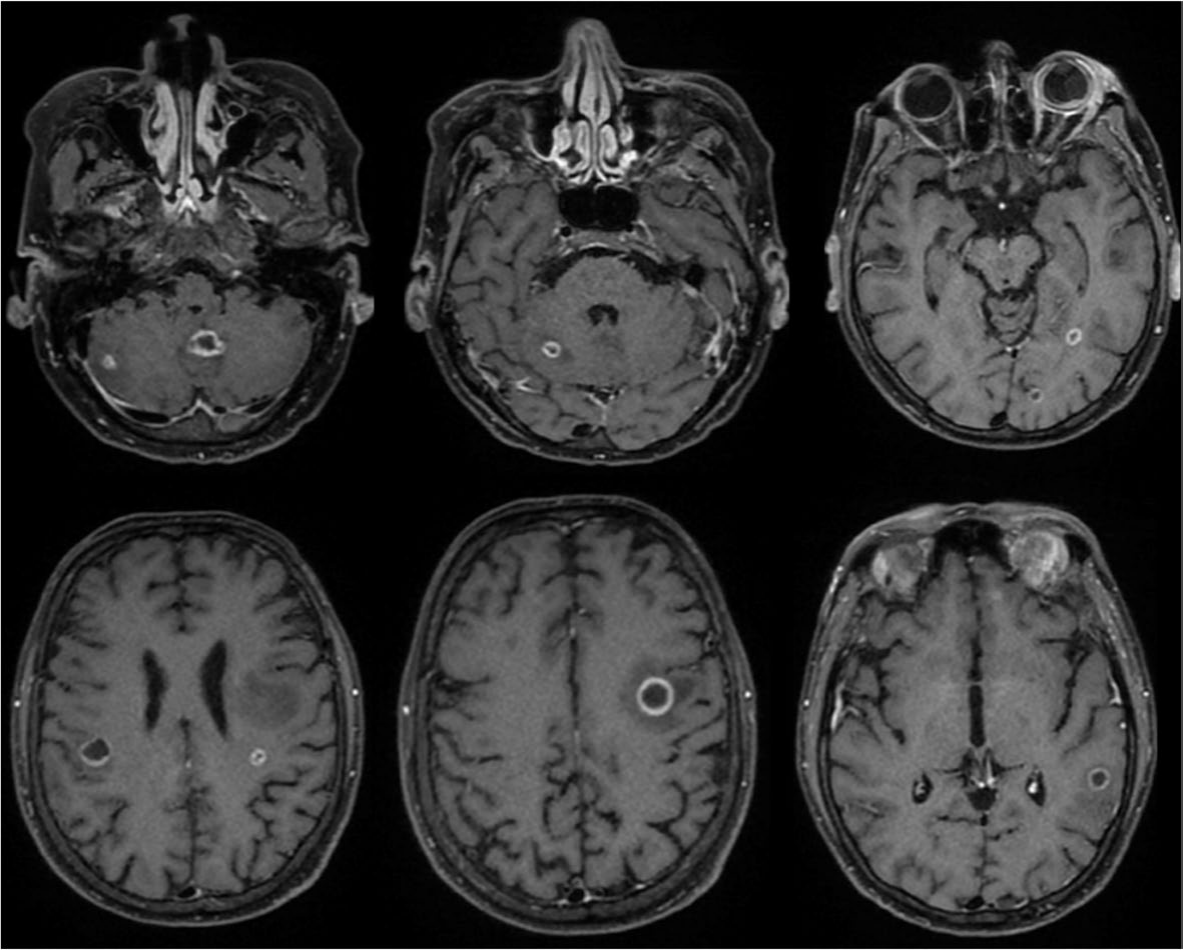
Contrast-enhanced T1-weighted images.* Multiple supra- and infratentorial rim-enhancing lesions. Also noted surrounding vasogenic edema. Findings that are consistent with abscesses. Images on the right show enhancement of the left globe and surrounding soft tissue. Relatively hyperintense subretinal fluid is noted. Findings that are consistent with left eye endophthalmitis and subretinal abscess. The right orbit shows enhancement of the extra-conal soft tissue suggesting involvement
